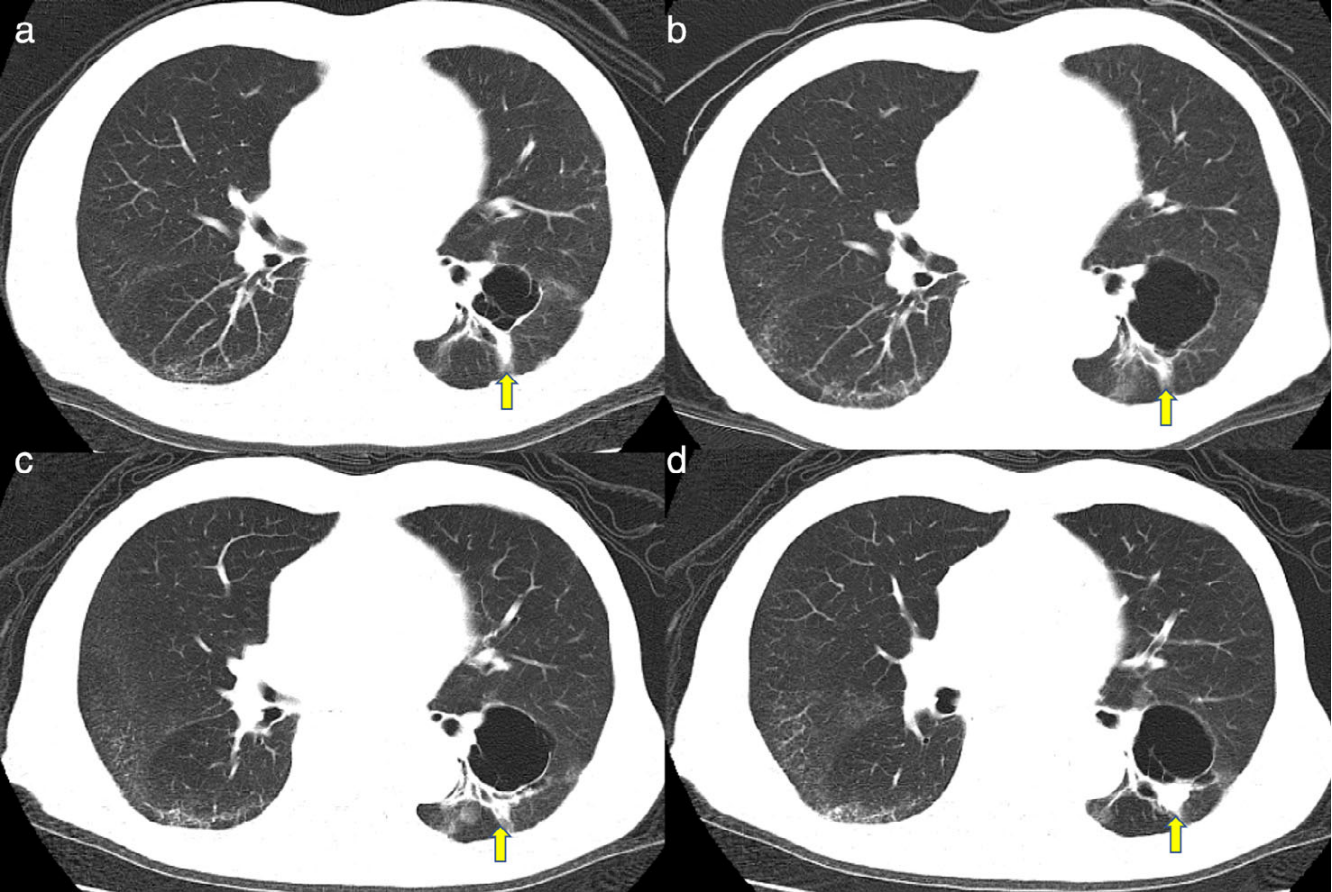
The clinical course of chest computed tomography (CT) findings. (a) The tumor shrunk three months after afatinib administration. (b) The antitumor effect of afatinib was maintained in partial response at 18 months and (c) 42 months after the start of afatinib treatment. (d) The tumor was enlarged 49 months after the initiation of afatinib. The yellow arrows indicate the primary tumor
